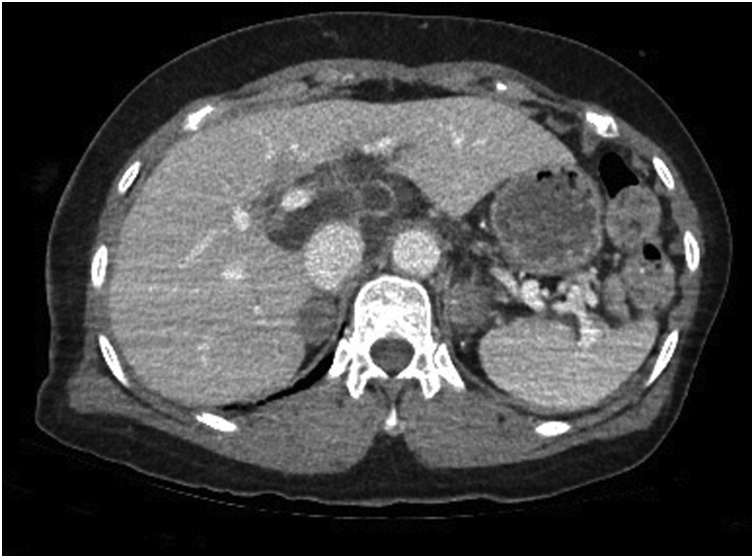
Axial view on an abdominal computed tomography scan of this patient, showing fluid collection and a cystic lesion around the caudate lobe of the liver.
